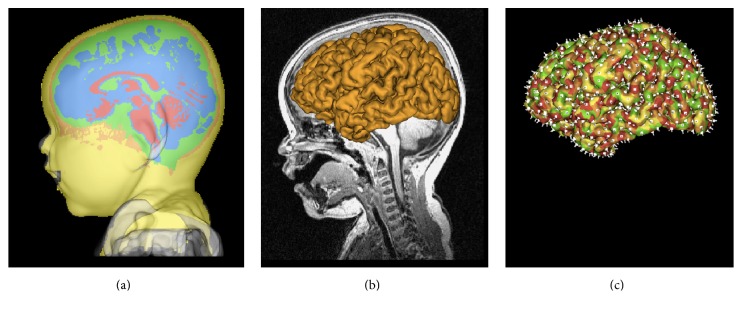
Electrical head model for an infant. (a) Registration of skull from CT with segmented MRI. (b) Cortical surface extraction overlaid with MRI. (c) Dipole tessellation of cortical surface for electrical source analysis.
